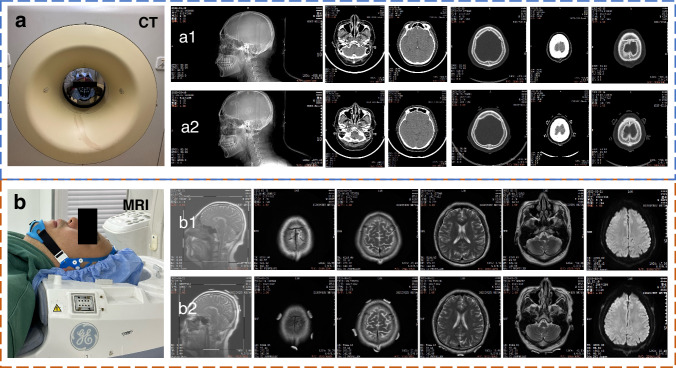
The CT and MRI of the brain. a a1 and a2 show the brain images of the subject undergoing CT scans with and without wearing hydrogel brain electrodes. b a1 and b2 present the brain images of the subject undergoing MRI scans with and without wearing hydrogel electrodes
